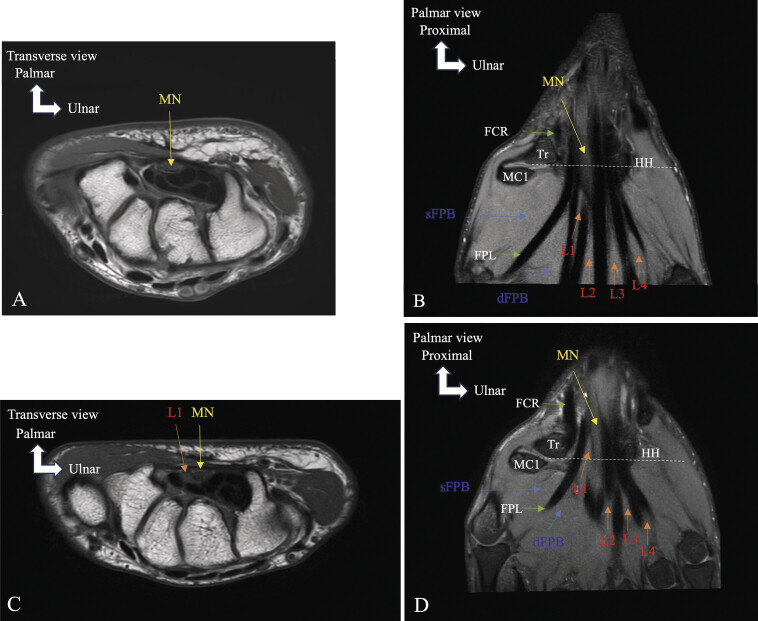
Proximal origin of the first lumbrical muscle within the carpal tunnel induces median nerve compression and carpal tunnel syndrome. Axial MRI in T1 TSE (
